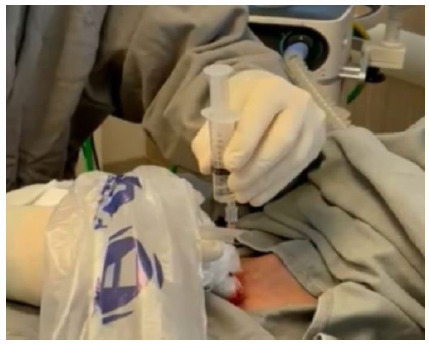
Centralized tracheal puncture with ultrasound guidance.
